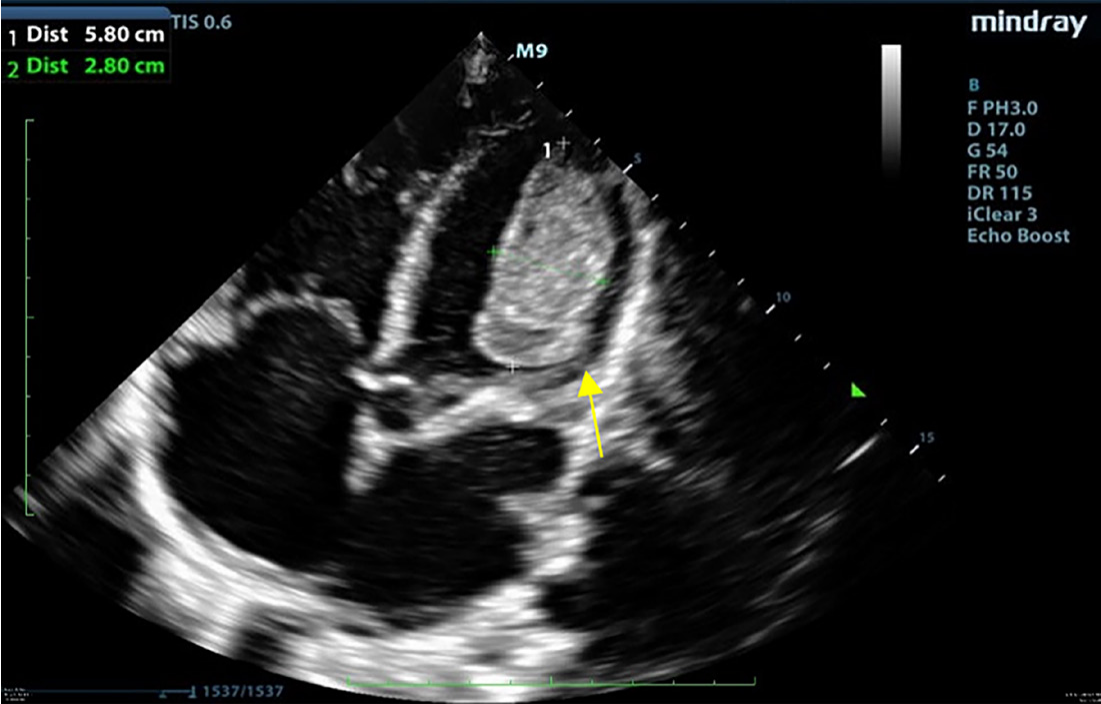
Apical four-chamber view of the heart showing a thrombus in the left ventricle measuring 5.8 × 2.8 centimeters (arrow).
